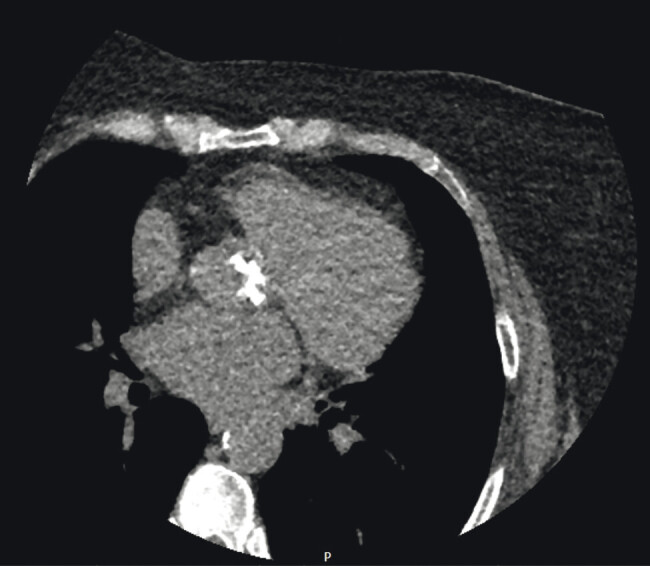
Cardiac CT coronary angiography at the level of the aortic valve demonstrating marked calcification of the bicuspid aortic valve.
